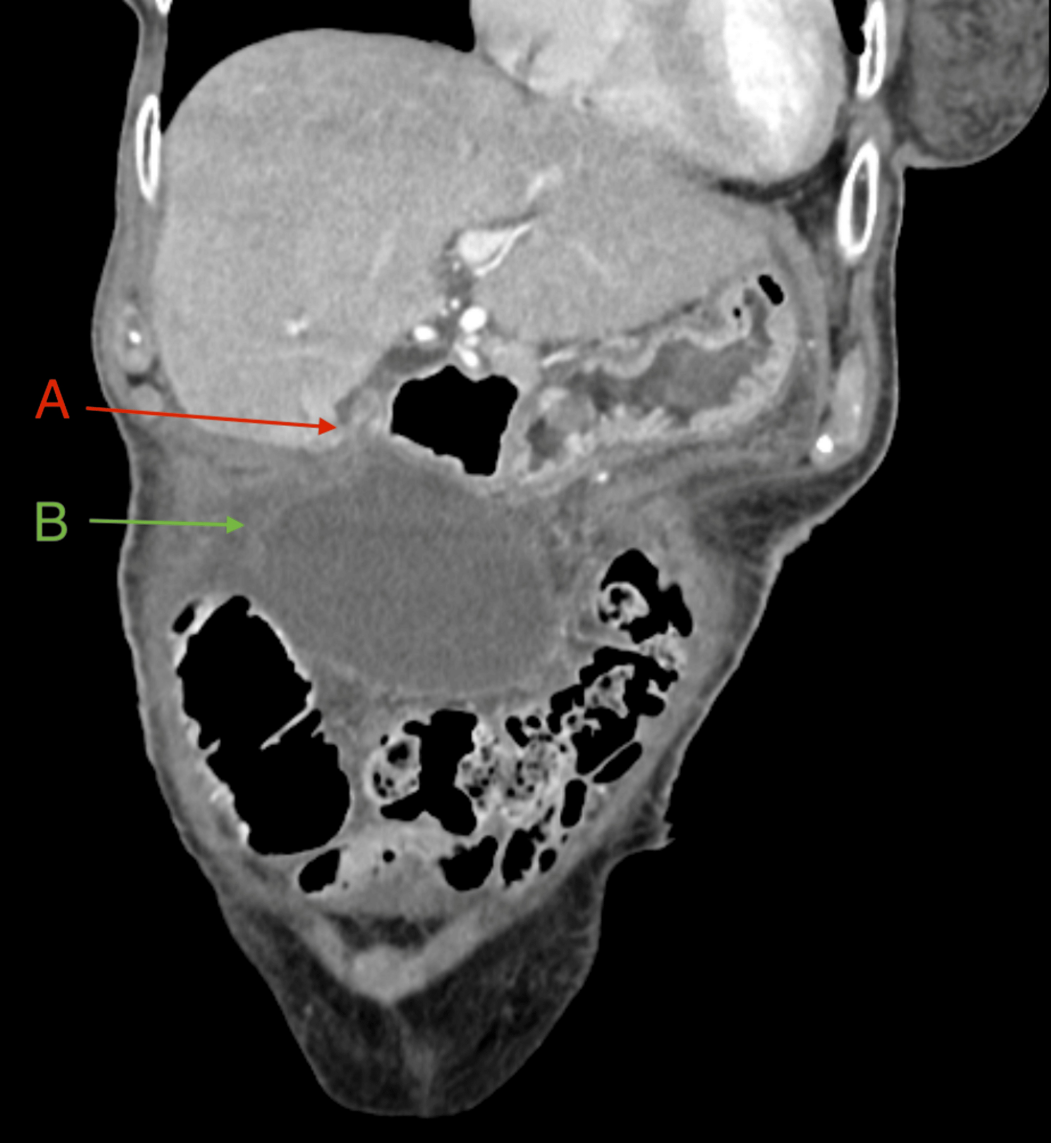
CT abdomen, coronal plane (early arterial phase). (A) Filling defect of the cystic artery (*arteria cystica*). (B) Gallbladder hydrops with pericystic fluid.
